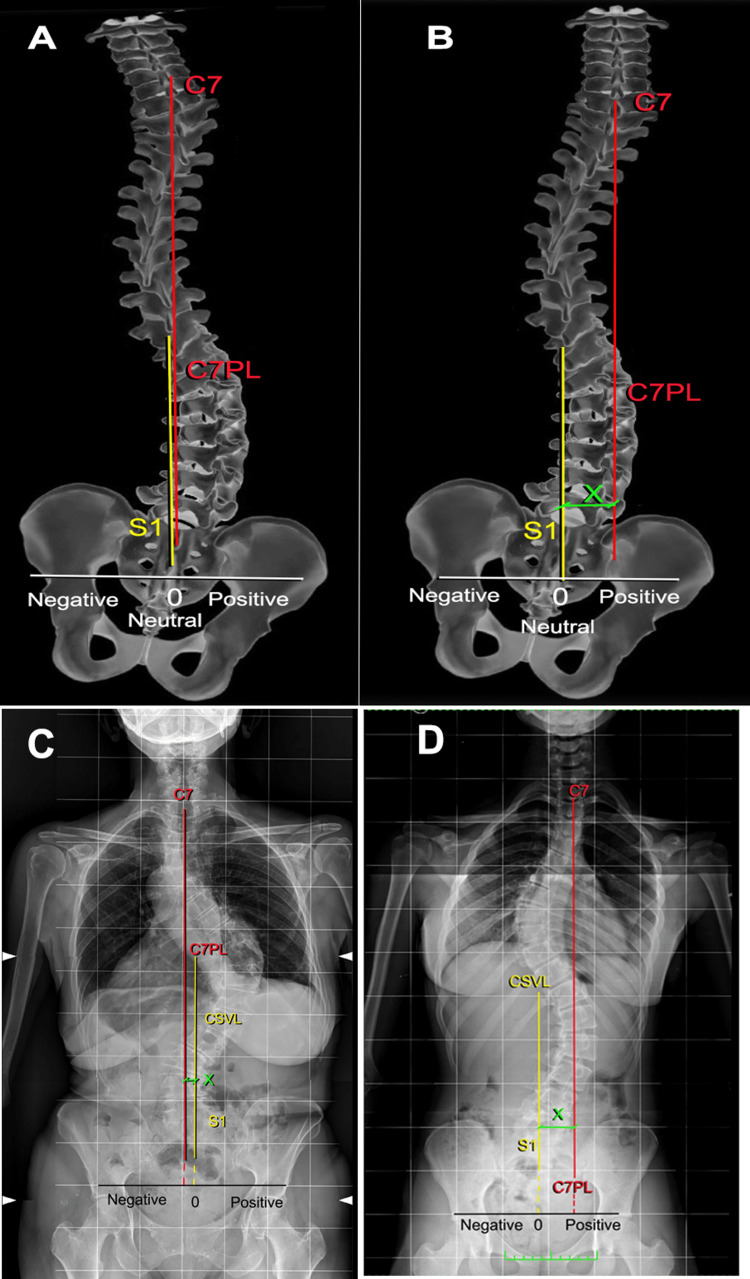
Example of the calculation of spinal balance on the coronal plane. Example of scoliotic spine with coronal compensation: A) scheme of scoliotic spine with coronal compensation; B) scheme of scoliotic spine with coronal decompensation; C) X-ray of a scoliotic patient with coronal compensation; D) X-ray of a scoliotic patient with coronal decompensation. Image Credit: Author Saverio Colonna
